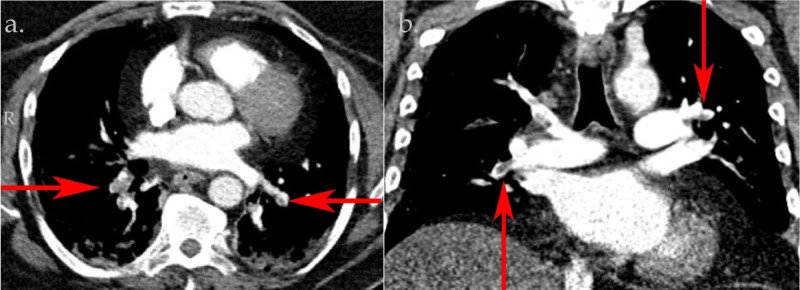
Pulmonary computed tomography angiography obtained on the day of hospital admission. Axial image (a) and coronal image (b) demonstrating bilateral filling defects of the pulmonary artery (red arrows).
